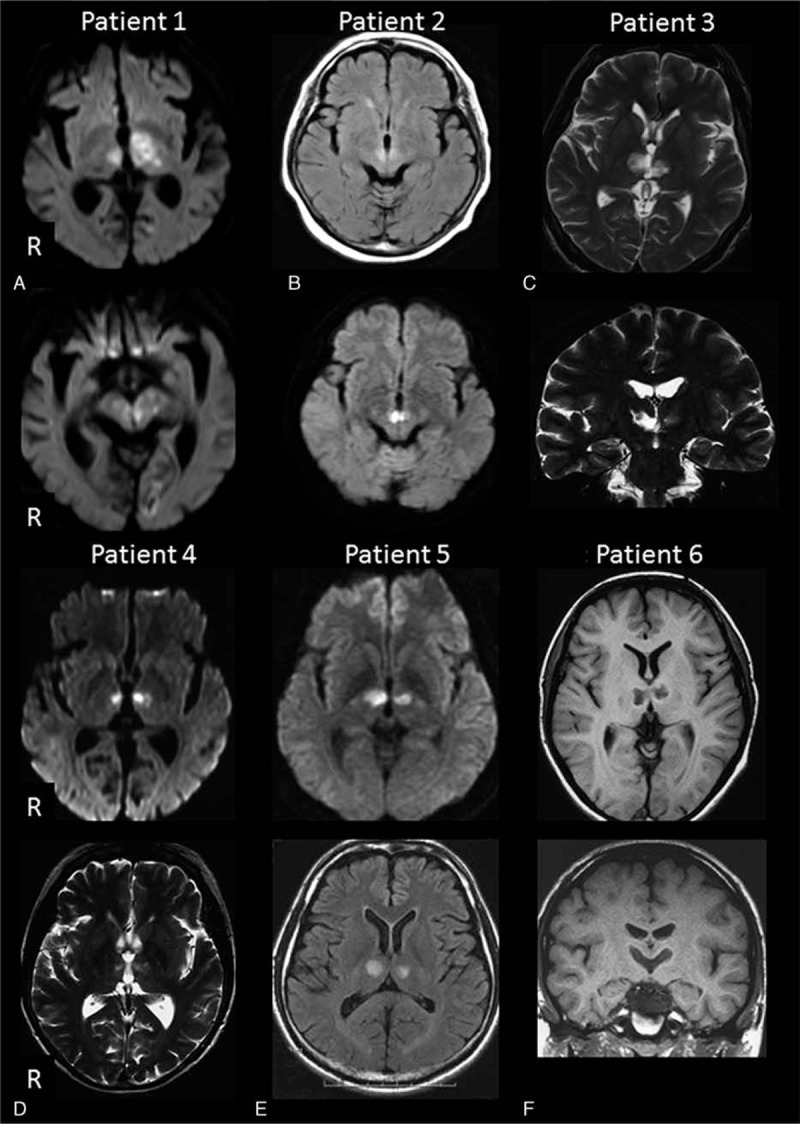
Magnetic resonance images of the patients with artery of Percheron infarctions. A, patient 1; B, patient 2; C, patient 3; D, patient 4; E, patient 5; F, patient 6.
